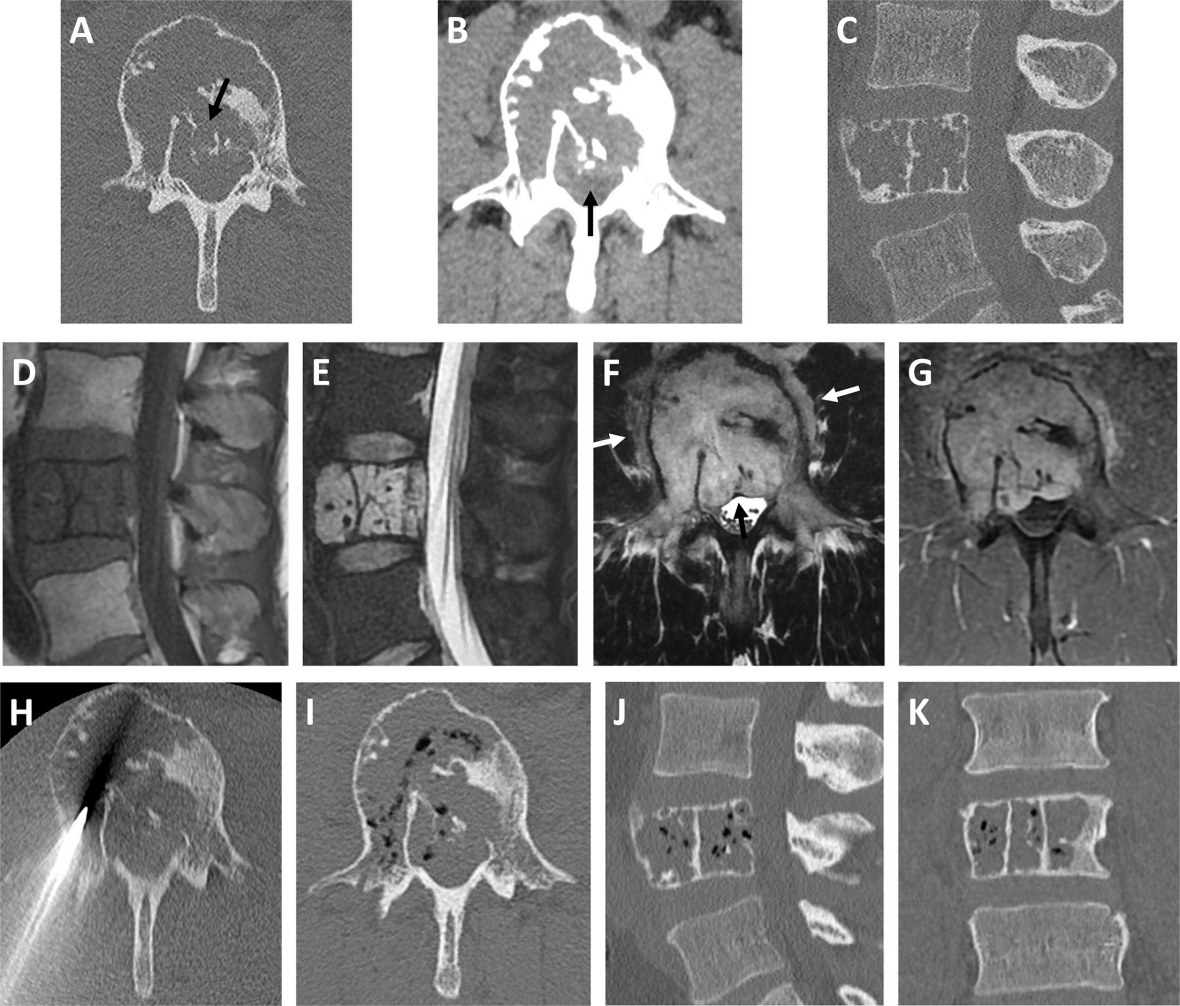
Expansile osteolytic lesion of the L4 vertebra, involving mostly the vertebral body with rupture of cortices in places (*arrow in A: axial slice in bone window CT-scan*)with posterior protrusion inside the spinal canal (*arrow in B: axial slice in soft tissue window CT-scan*). The lesion was composed of thickened vertical trabeculae (*C: sagittal reconstruction in bone window*). MRI showed a low signal on *T*_1_ weighted images (*D: sagittal slice*)and high signal on STIR-weighted images (*E: sagittal slice*), with invasion of the surrounding soft tissue and posterior protrusion inside the spinal canal (*arrows in F: axial T_2_ weighted slice*). The lesion was strongly and homogeneously Gadolinium-enhanced *(G: axial T_1_ weighted contrast-enhanced slice)*. After CT-guided biopsy *(H)*, gas bubbles have formed along the biopsy path and inside the lesion, notably in its epidural component *(I)*. The diffusion of gas bubbles inside the vertebral body is also shown in sagittal *(J)* and coronal *(K)* reconstructions. STIR, shorttau inversion recovery.
